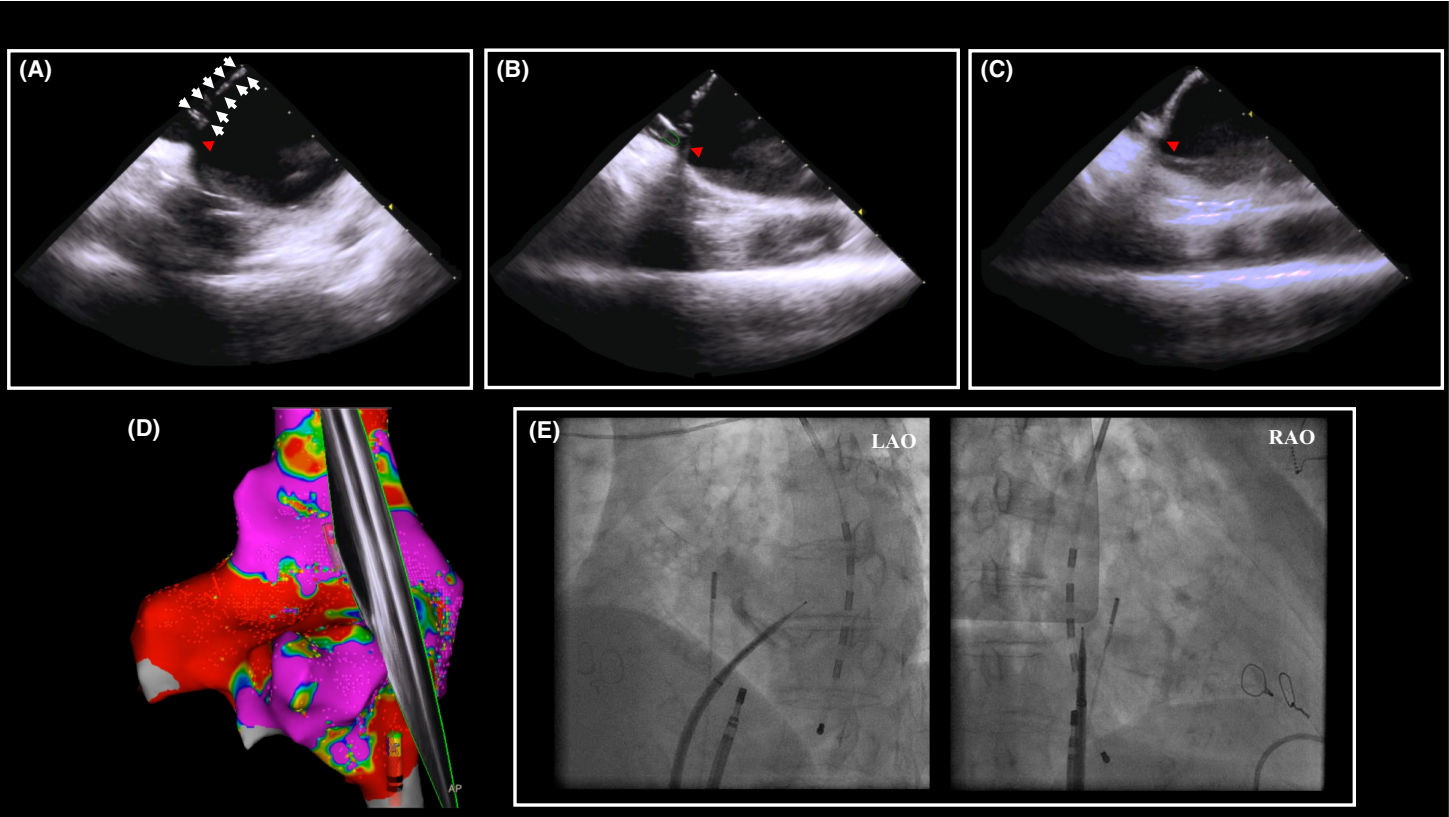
(A) Intracardiac echography revealed a small channel (arrow) with a surrounding artificial patch (arrowhead). (B) The native septum was marked using the ablation catheter based on the ultrasound image with an intracardiac magnetic sensor (arrow). (C) An SL‐0 sheath was placed on the exact location of the residual atrial septum (arrow). (D) An right atrial voltage map and the direction of the ultrasound image recorded by a SOUND STAR catheter is shown. (E) The transseptal puncture succeeded with a radiofrequency needle.
